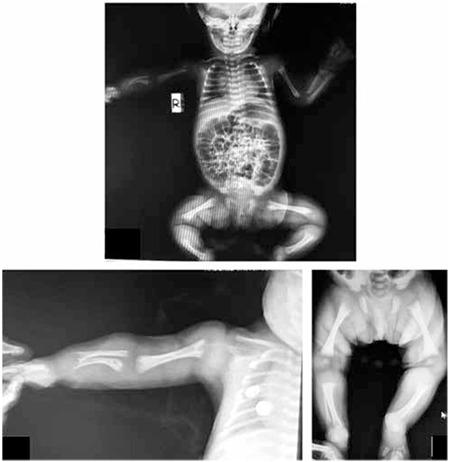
Radiographic imaging of the patient showing distorted trabeculation, reduced mineralization, metaphyseal irregularities, cupping, diaphyseal shortening, shortness of the right humerus, ulna, and radius
